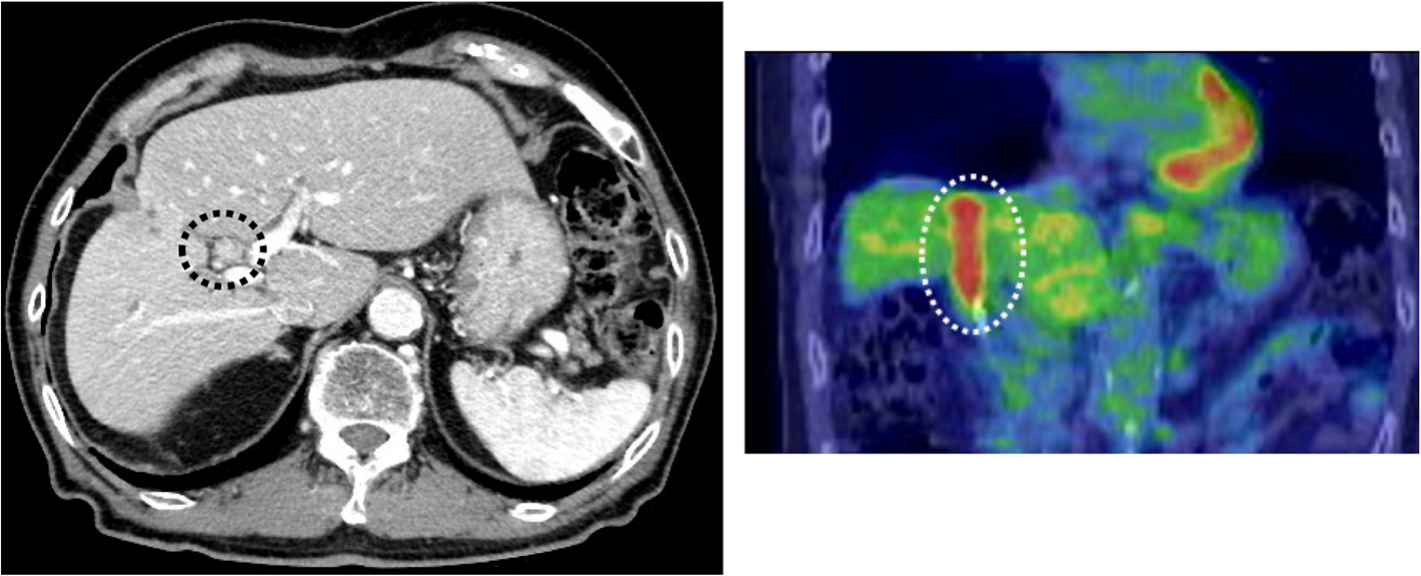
Computed tomography (axial image, black-dotted line circle) and PET–CT (coronal image, white-dotted line circle) revealed an intrahepatic bile duct tumor with the accumulation of FDG
